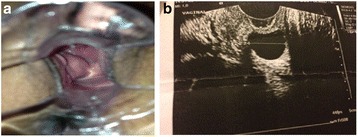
a Cystic lesion in the right wall of the vagina close to the fornix. b Ultrasound showed a thin-walled cyst with anechoic content on the right posterolateral wall of the vagina
